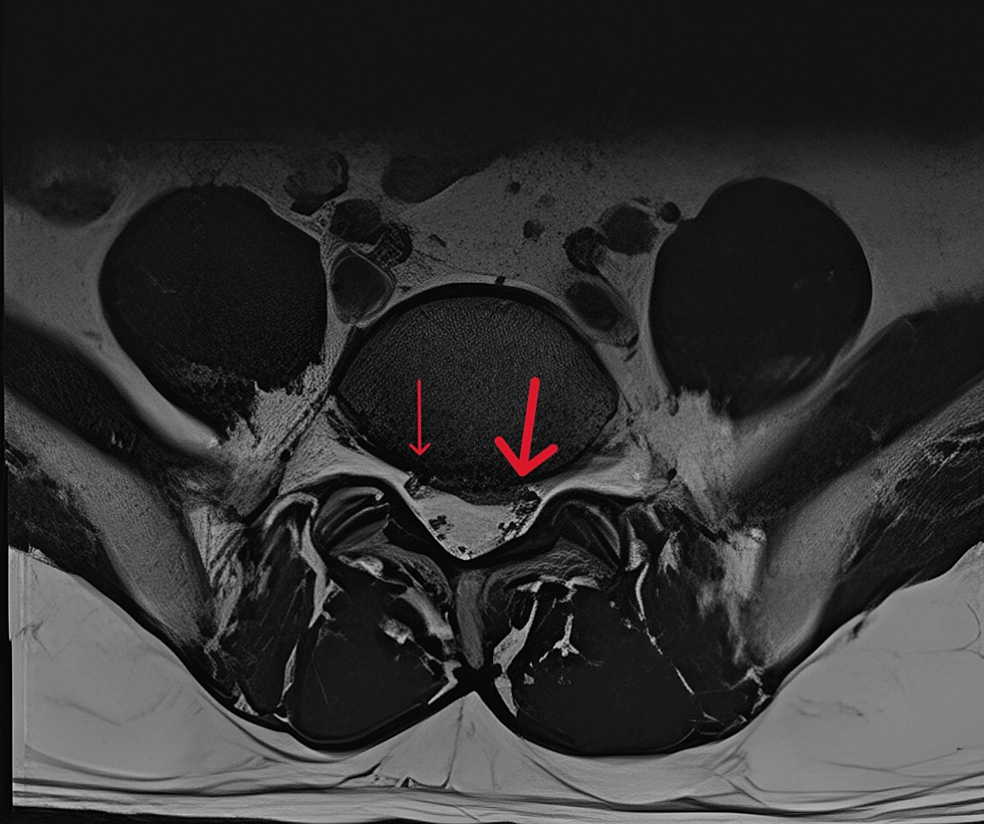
Axial view of T2-weighted MRI, with a small red arrow indicating grade 1 stenosis where the disc is in contact with the nerve root without deviation, while a large red arrow shows grade 2 stenosis with evident deviation of the nerve root
